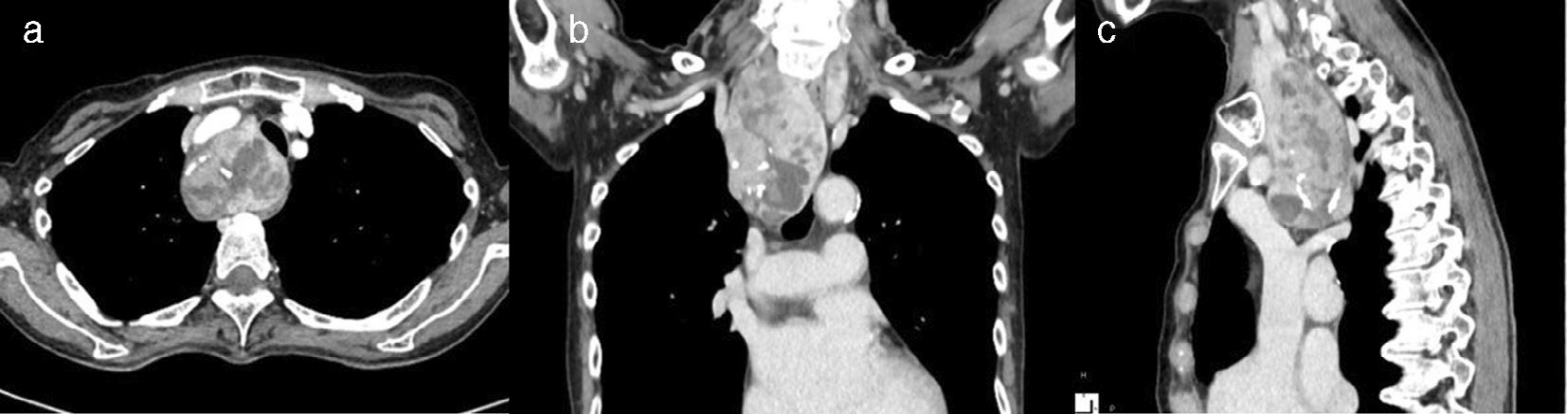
Chest computed tomography showed the trachea stenosed by the goiter (a). Coronal (b) and axial (c) sections showed the tumor extending beyond the level of the carina
